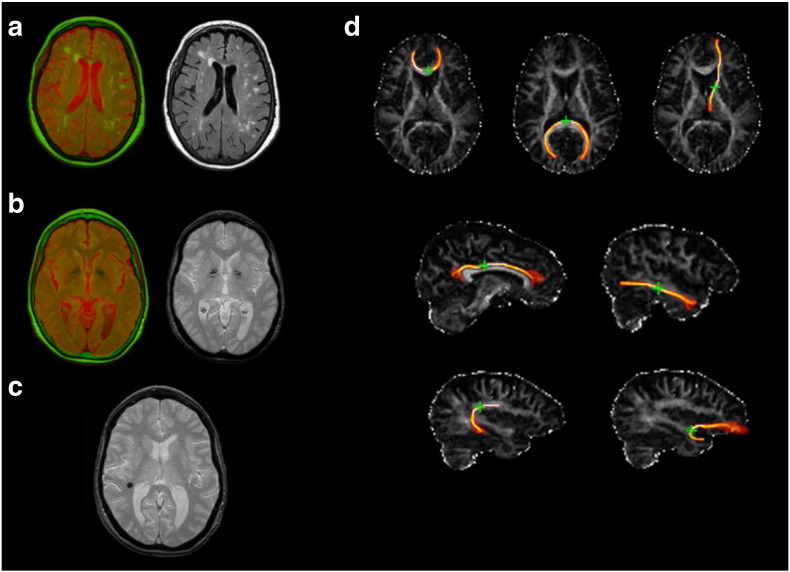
Example images of brain MRI features measured. (a) White matter hyperintensities (WMH): fused T2*W and FLAIR images mapped in red and green colour showing WMH in light green, left, and original FLAIR image, right. (b) Iron deposits: fused T2*W and FLAIR images mapped in red and green colour, showing iron deposits in dark green, left, and original T2*W image, right. (c) Microbleed (in black) as seen in T2*W. (d) FA maps with examples of segmented white matter tracts, with green cross indicating the seed point: genu and splenium of the corpus callosum and anterior thalamic radiation (upper row); rostral cingulum and inferior longitudinal fasciculus (middle row); arcuate and uncinate fasciculi (lower row).
